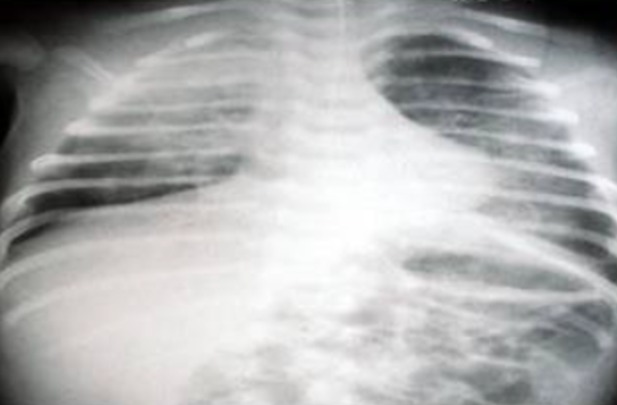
Radiographie du thorax de face de l’observation n°4 montrant une pneumopathie bilatérale (apicale droite et basale gauche)
